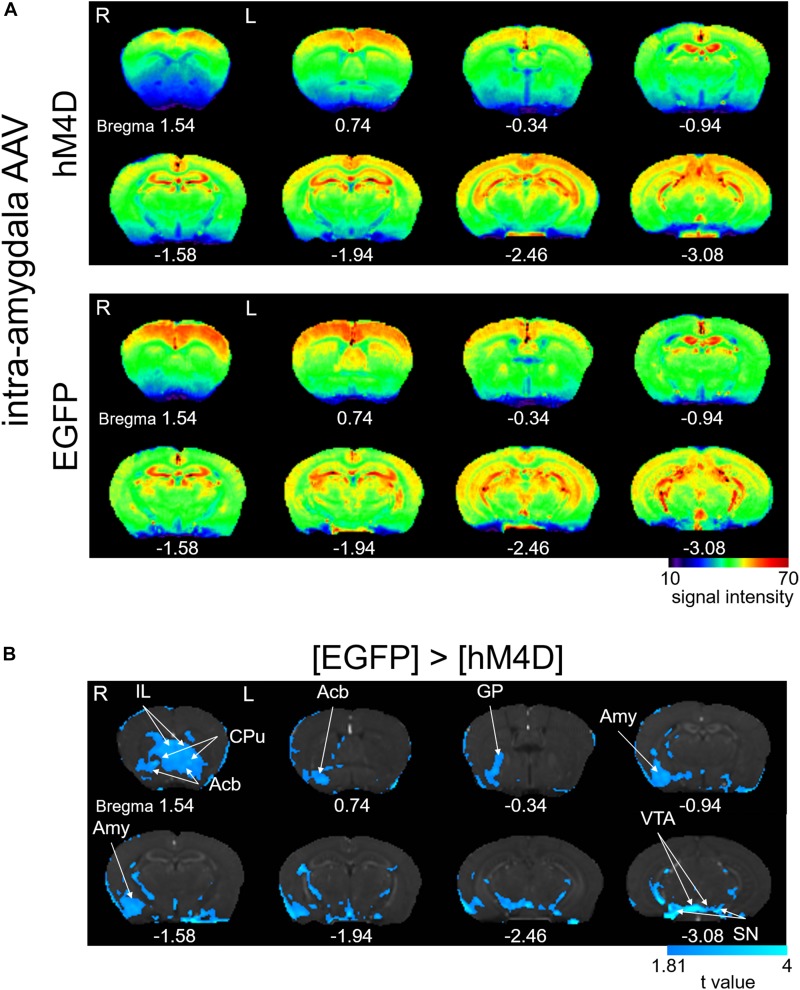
Effects of repeated activation of hM4D receptors expressed in the right amygdala on manganese accumulation. (A) Color map display of averaged T1-weighted images in [hM4D] and [EGFP]. R, the right side; L, the left side (for all panels in this figure). The color bar indicates normalized signal intensity. The numbers below the images indicate the anterior-posterior coordinate from bregma. (B) Comparisons of the signal intensity between [hM4D] and [EGFP] shown as *t*-value plots (*P* < 0.05, cluster-wise FDR corrected). IL, infralimbic cortex; Acb, nucleus accumbens; GP, globus pallidus; Amy, amygdala; VTA, ventral tegmental area; SN, substantia nigra. The color bar indicates the *t*-value (the signals with [hM4D] were significantly smaller than those with [EGFP]).
